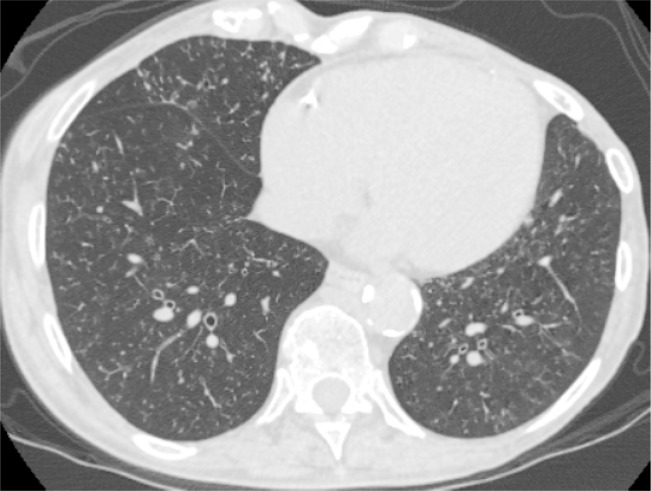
HRCT scan of the chest, showing diffuse micronodules and tree-in-bud opacities.
